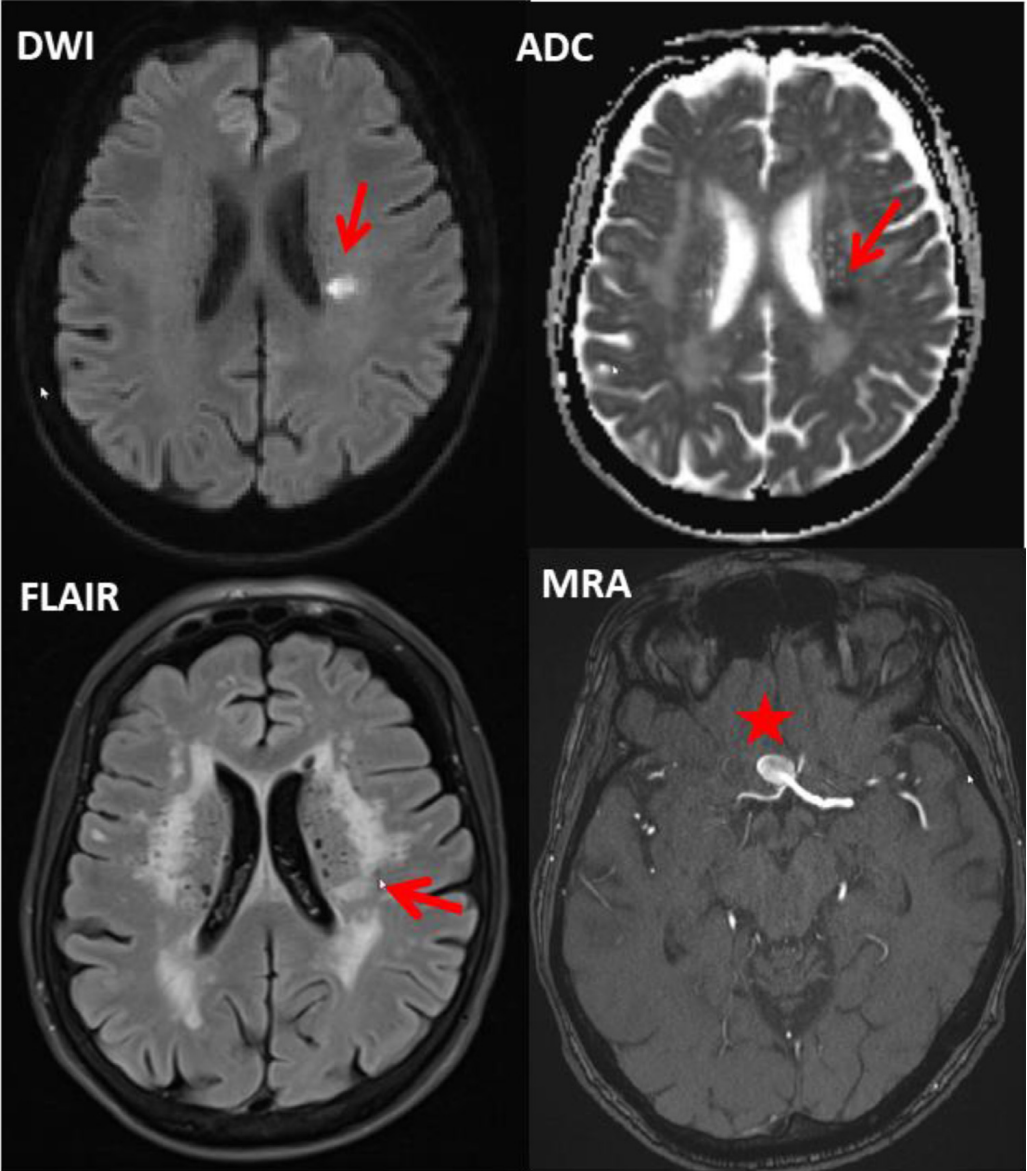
Cerebral MRI of the index patient showing an acute ischemic lesion periventricularly on the left side (arrows), leucoaraiosis, and an aneurysm of the communicant anterior artery (asterik) with 10 mm in diameter (DWI, Diffusion Weighted Imaging; ADC, Apparent Diffusion Coefficient; FLAIR, Fluid Attenuated Inversion Recovery; MRA, MR-Angiography).
